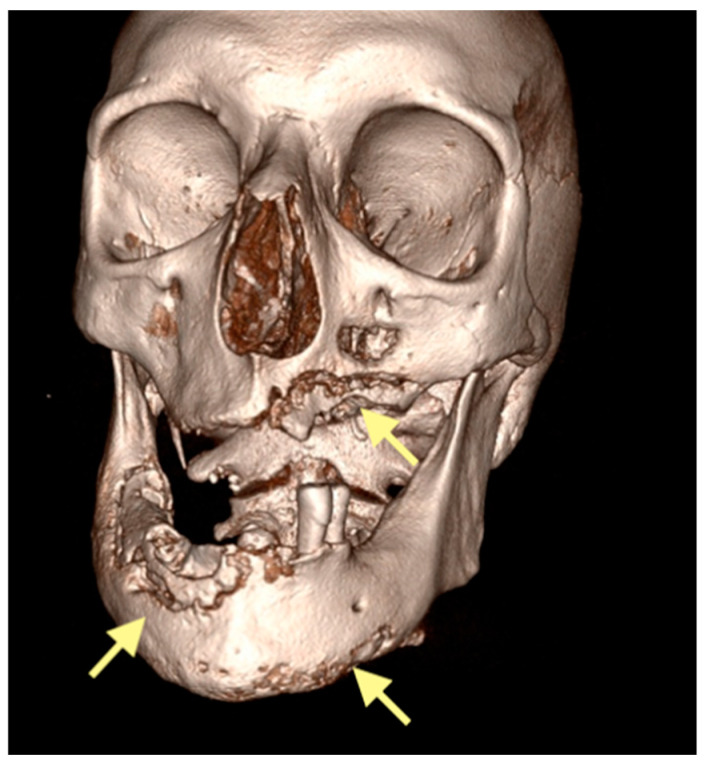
CT of the head and facial bones (16 December 2024).
